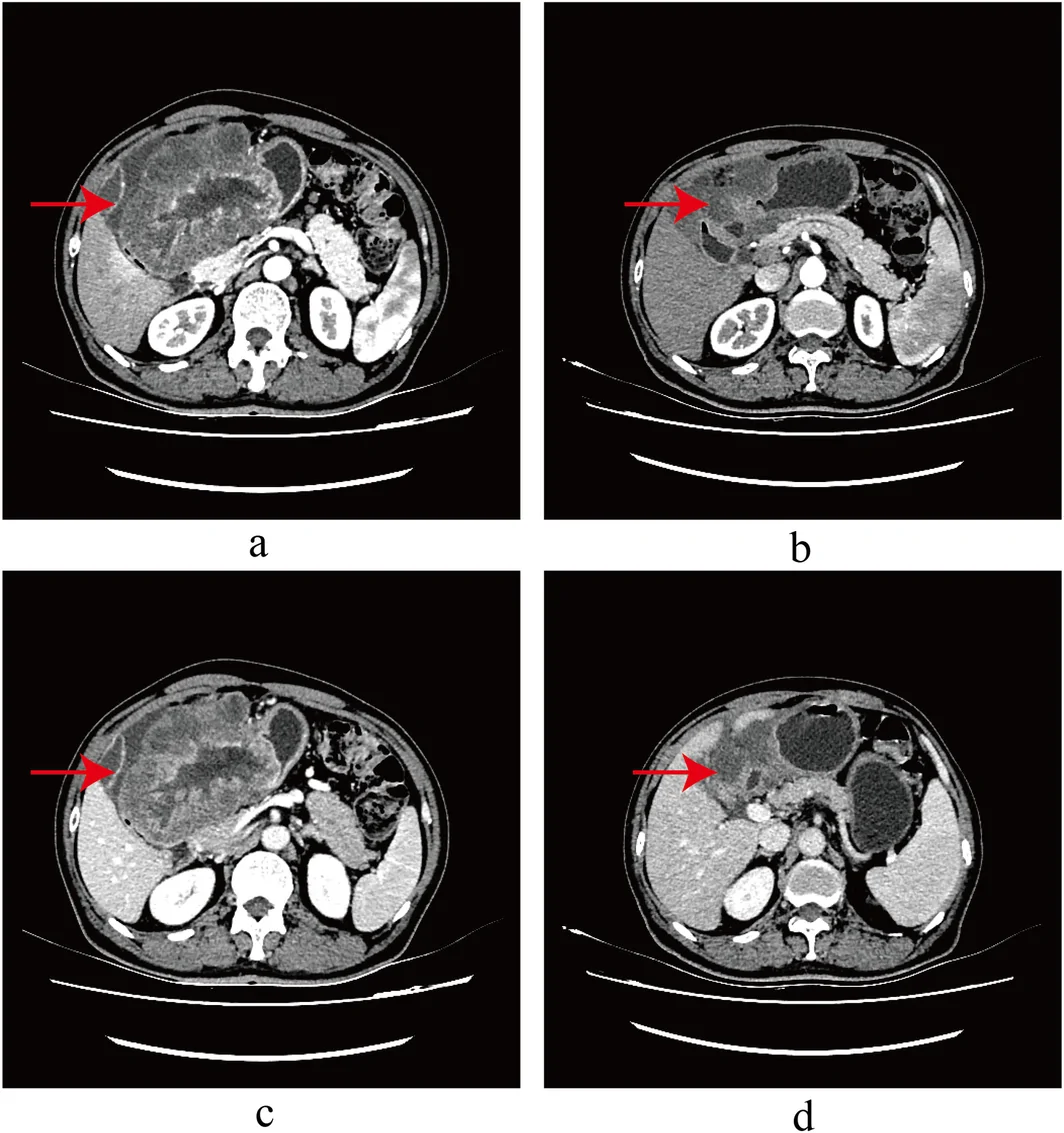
Enhanced abdominal computed tomography images for evaluating the treatment response. a: The arterial phase CT radiomics on July 18, 2023; b: The arterial phase CT radiomics on February 27, 2024; c: The venous phase CT radiomics on July 18, 2023; d: The venous phase CT radiomics on February 27, 2024.
